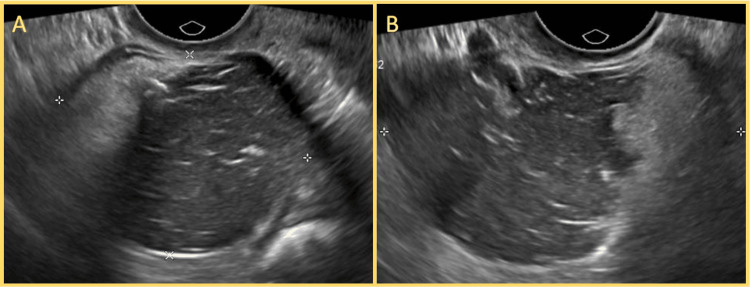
Transvaginal ultrasound of the left adnexal mass (A, B) Longitudinal views showing a complex cystic lesion in the left ovary, approximately 3 cm in diameter. The mass exhibits heterogeneous internal echoes, thin septations, and irregular walls. These sonographic features were suggestive of a complex ovarian cyst and prompted further evaluation by laparoscopy and left adnexectomy.
